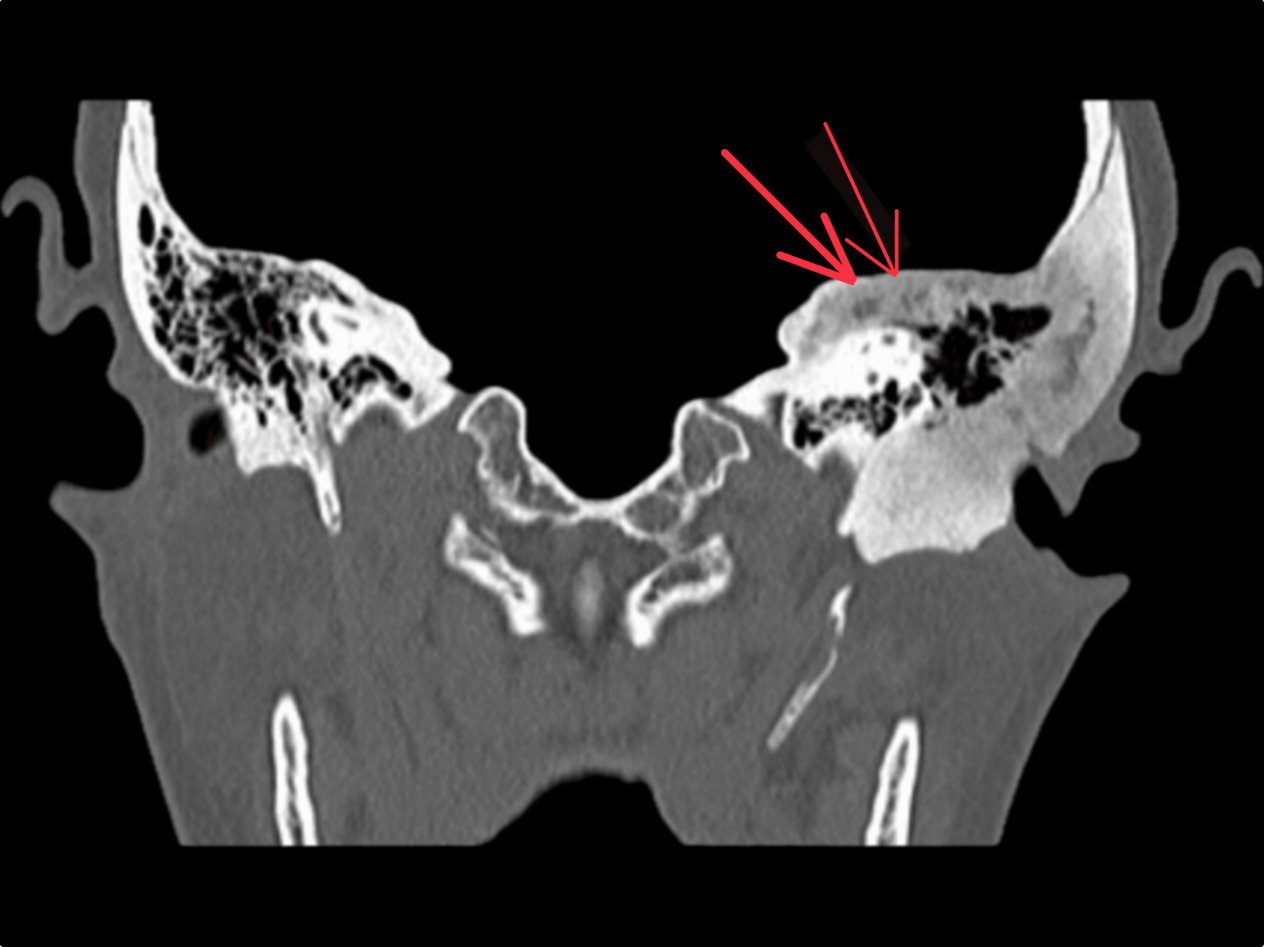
CT Temporal Bones, Coronal View Coronal CT of the temporal bones showing fibrous dysplasia in the petrous and squamous portions of the left temporal bones (red arrows).
